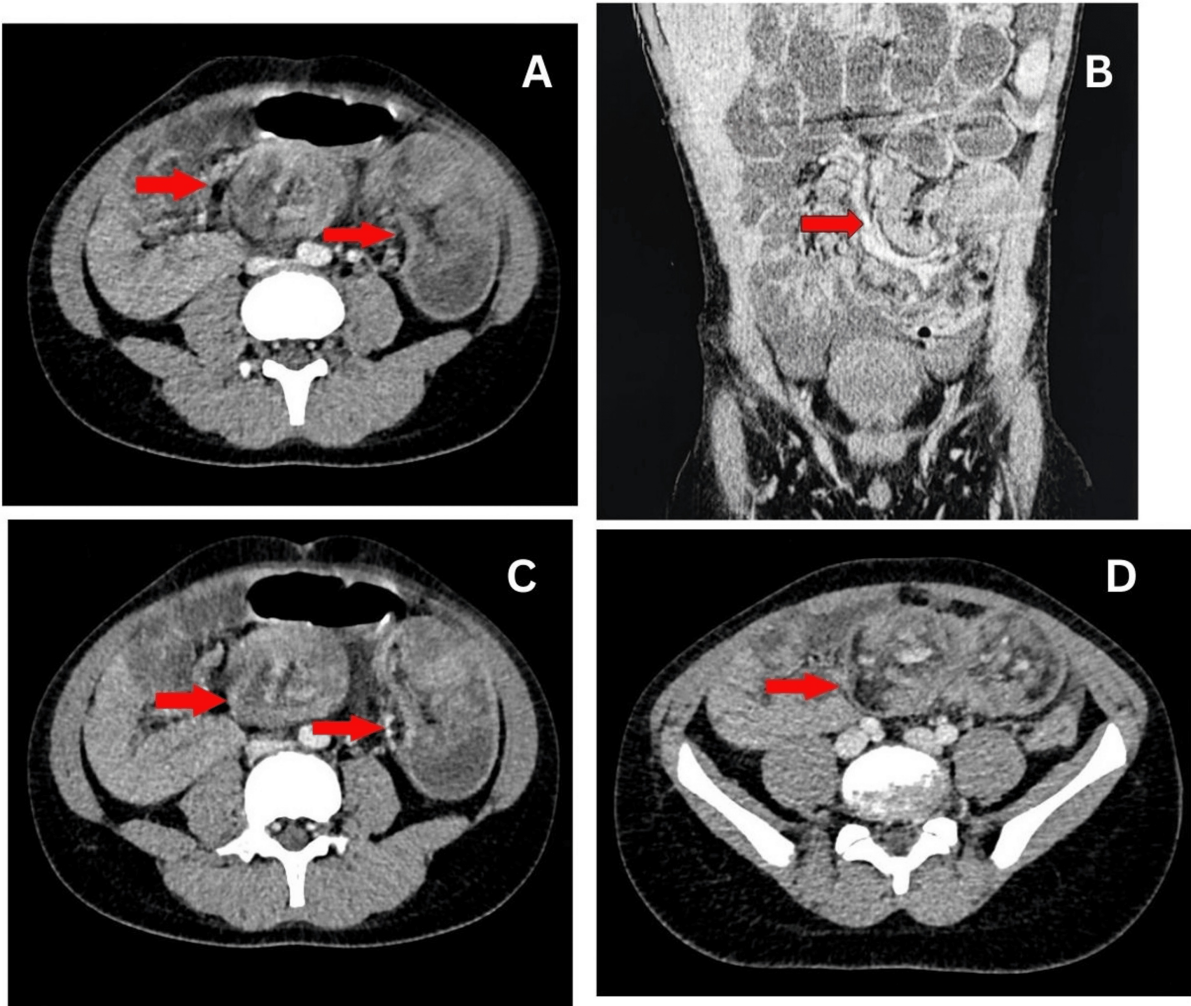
Computer tomography (CT) imaging A: axial image showing the “target sign” of intussusception with mesenteric lymphadenopathy (arrows); B: axial view demonstrating intussusception and a “whirlpool sign” consistent with midgut volvulus (arrow); C: axial image again showing the target sign (arrows); D: axial image highlighting enlarged mesenteric lymph nodes within the intussuscepted segment (arrow).
